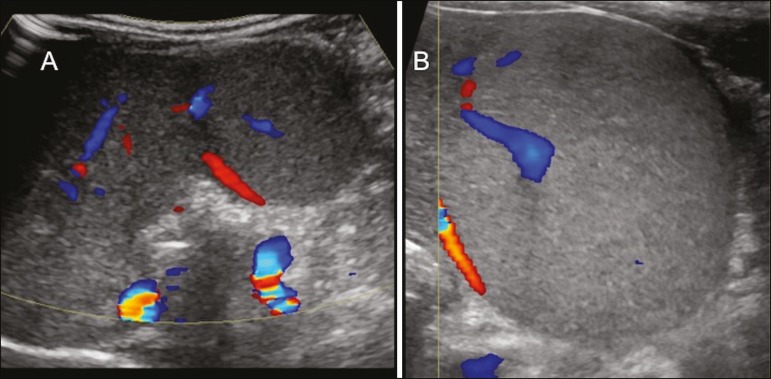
Liver regeneration nodule in a 3-month-old girl diagnosed with biliary atresia. Abdominal ultrasound with convex and linear transducers (A and B, respectively), showing a large, well-defined hyperechoic nodule in the left hepatic lobe. The image in B shows that there is no vascular distortion, and a portal branch can be seen within the lesion. Surgical resection was performed, and the pathology study indicated a regeneration nodule.
